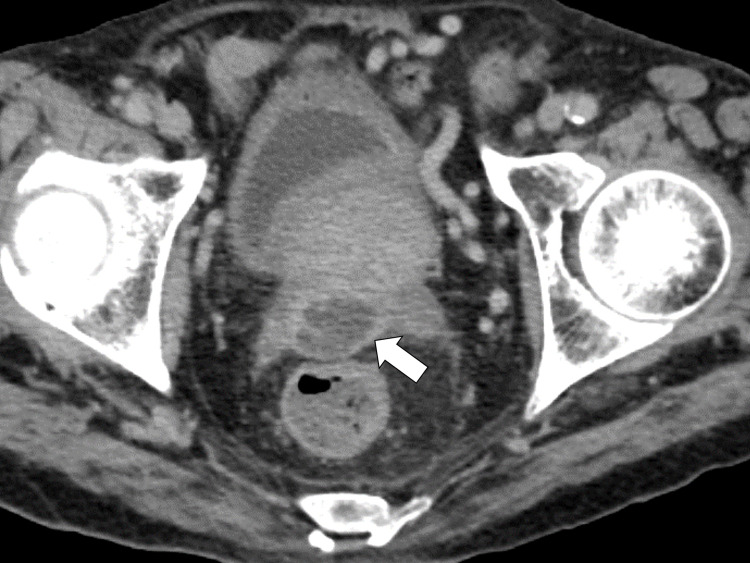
Pelvic computed tomography image showing fluid retention in the posterior part of the prostate.
